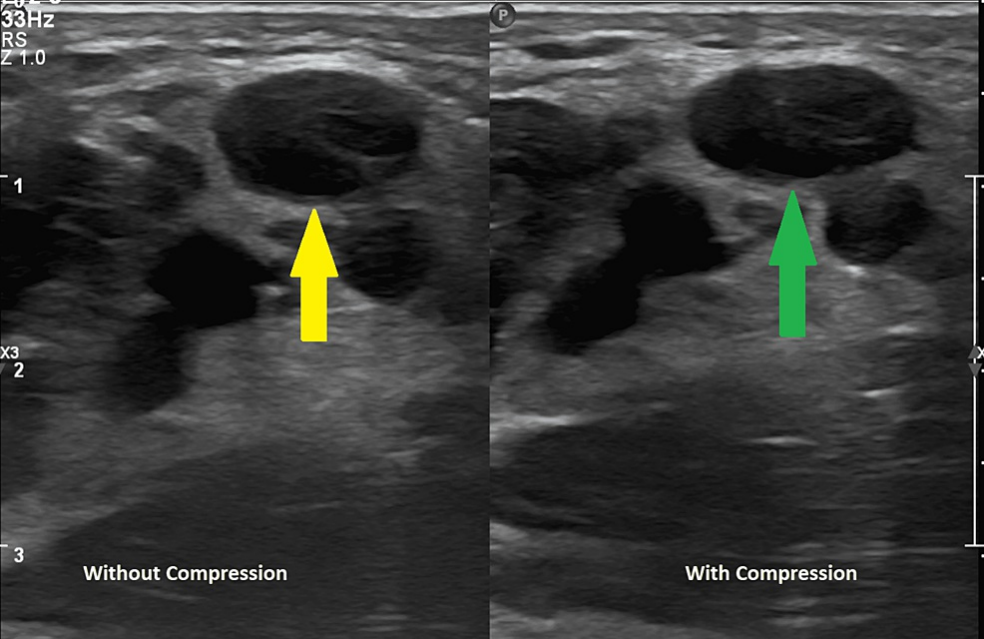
Ultrasound showing a non-compressible right axillary vein (yellow arrow: right axillary vein without compression; green arrow: with compression).
